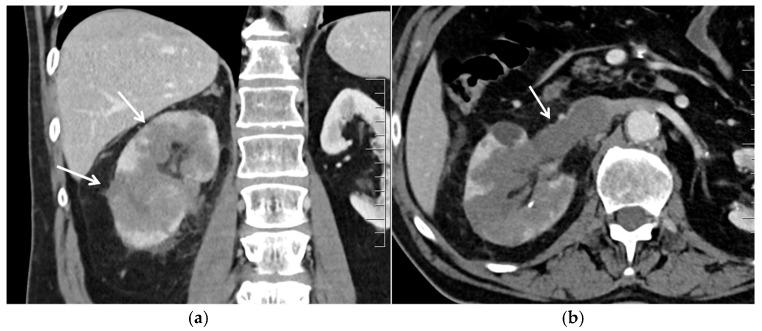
Infiltrating right clear cell RCC with renal vein thrombosis. (a) Contrast-enhanced coronal CT image in the venous phase demonstrates a hypoenhancing infiltrative lesion (arrows) with an ill-defined interface with the adjacent normal renal parenchyma. The reniform contour of the kidney is preserved. (b) Contrast-enhanced axial CT image in the venous phase shows tumor extension into the right renal vein (arrow), reaching the inferior vena cava.
